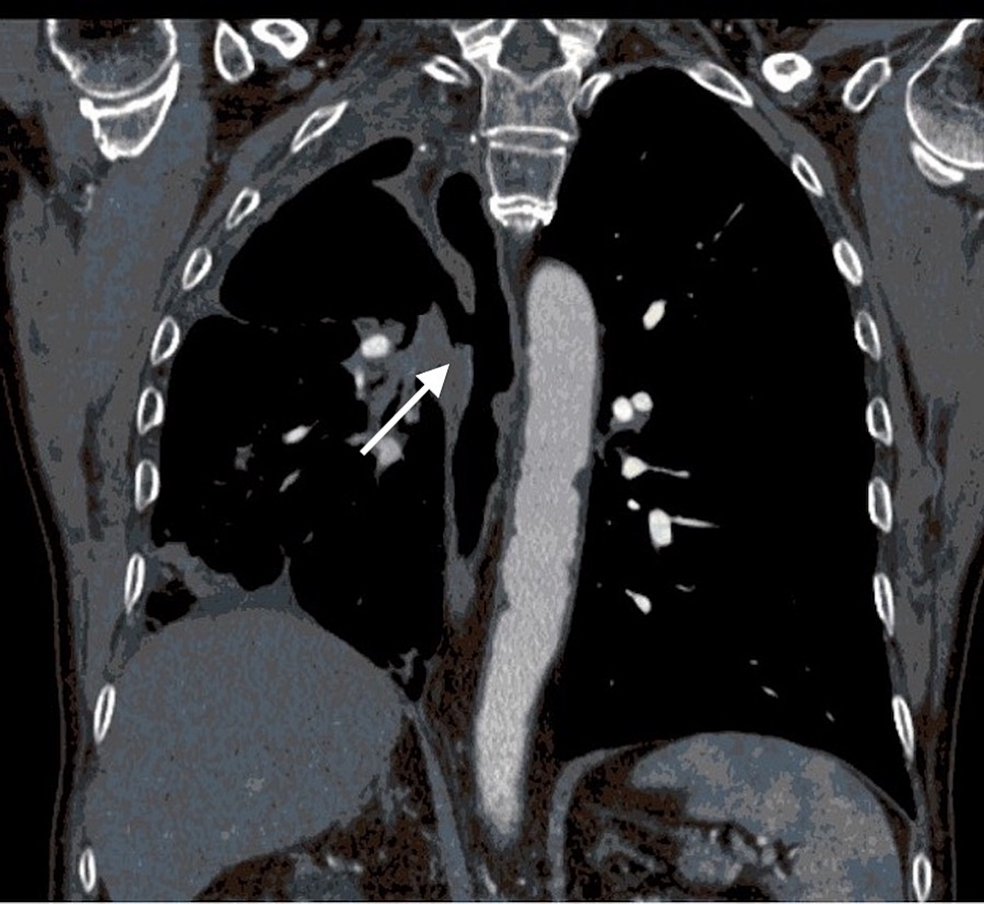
Coronal view of the computed tomography image of the parenchymal-esophageal fistula before closure (arrow)
